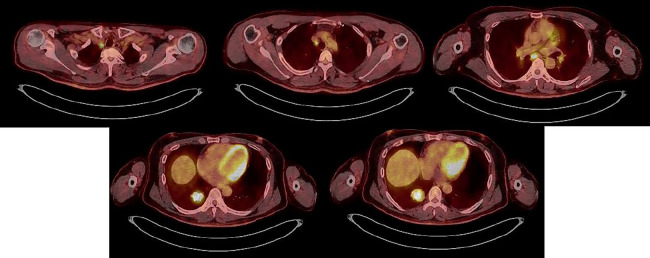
PET-CT showed the accumulation for a mass lesion (SUV max 12.64) in the right lower lobe S10
